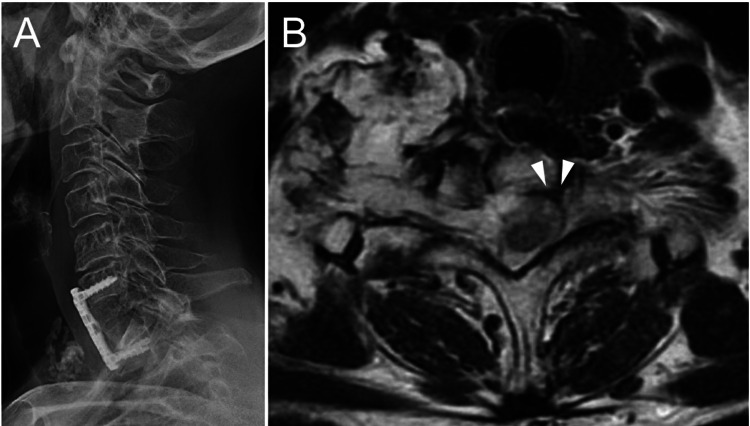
Postoperative lateral radiograph and T2-weighted imaging (A) Postoperative lateral radiograph showing anterior cervical fusion with a plate at the level of C6-T1 with autologous iliac bone grafting. (B) T2-weighted axial imaging revealing complete resection of the tumor in the left C7-T1 foramen (arrowhead) and residual tumor in the right C7-T1 foramen.
